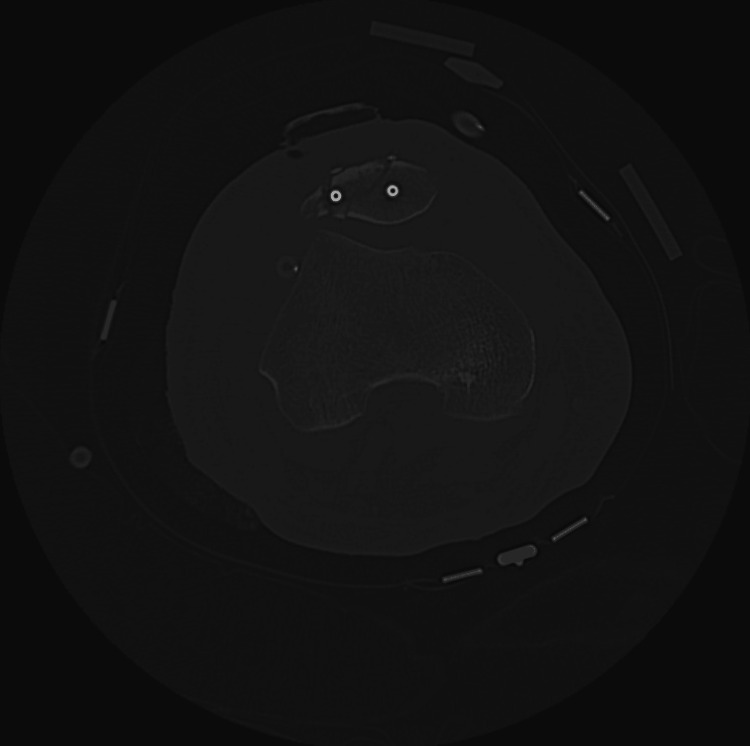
Computed tomography of the right knee showing nonspecific swelling and fluid collection in the anterior knee.
